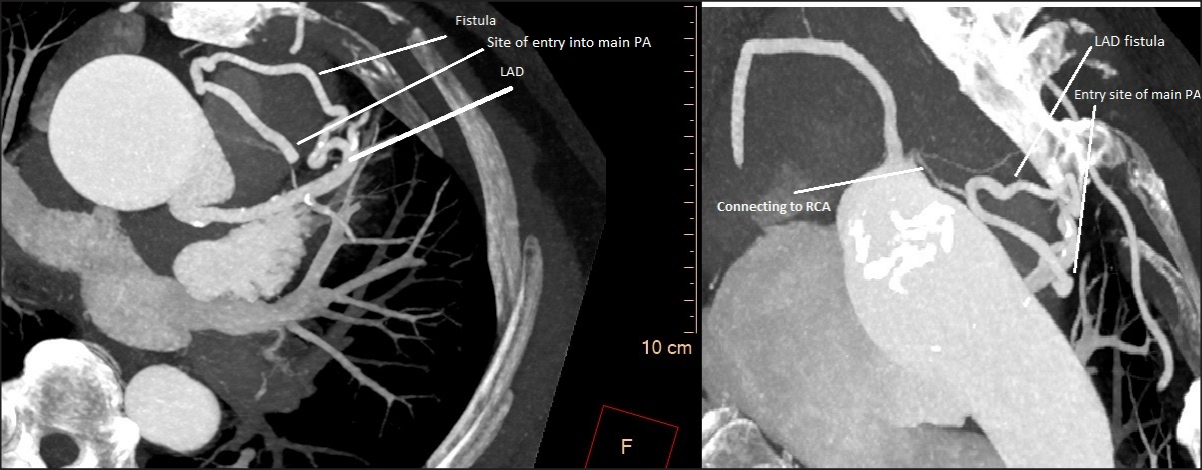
A coronary computed tomography angiography axial view showing the course of the fistula.
